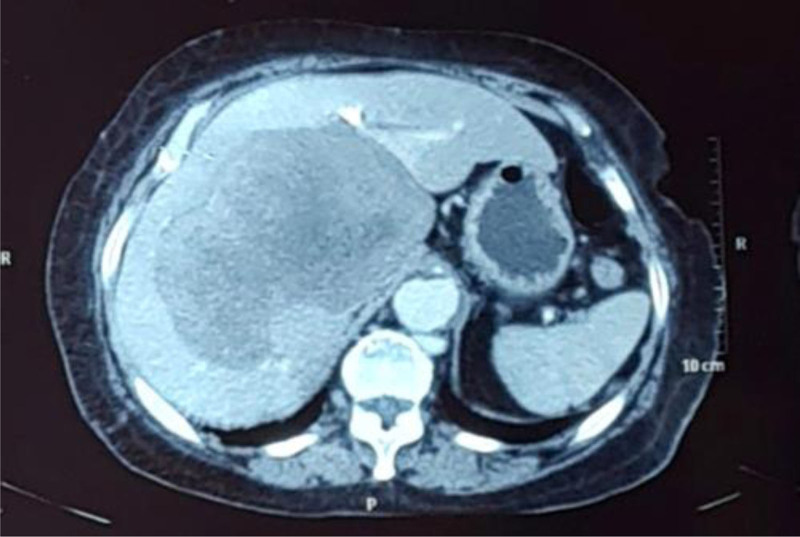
Computed tomography axial view (arterial phase) showing a large, hypodense lobulated mass involving segment IV, VII and VIII of the liver.
